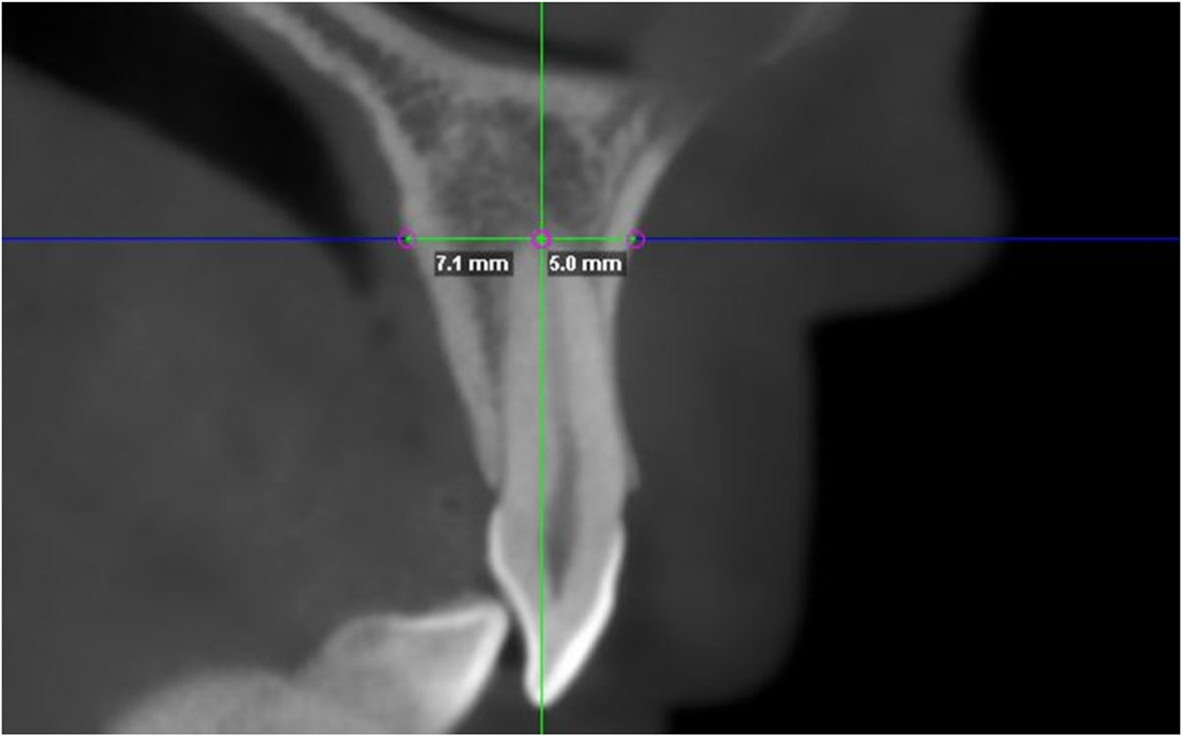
Measurement of the alveolar bone thickness at apical area of lateral incisors in CBCT. In the sagittal plane, alveolar bone thickness was evaluated in a bucco-lingual direction perpendicular to the long axis of each tooth at the root apex
